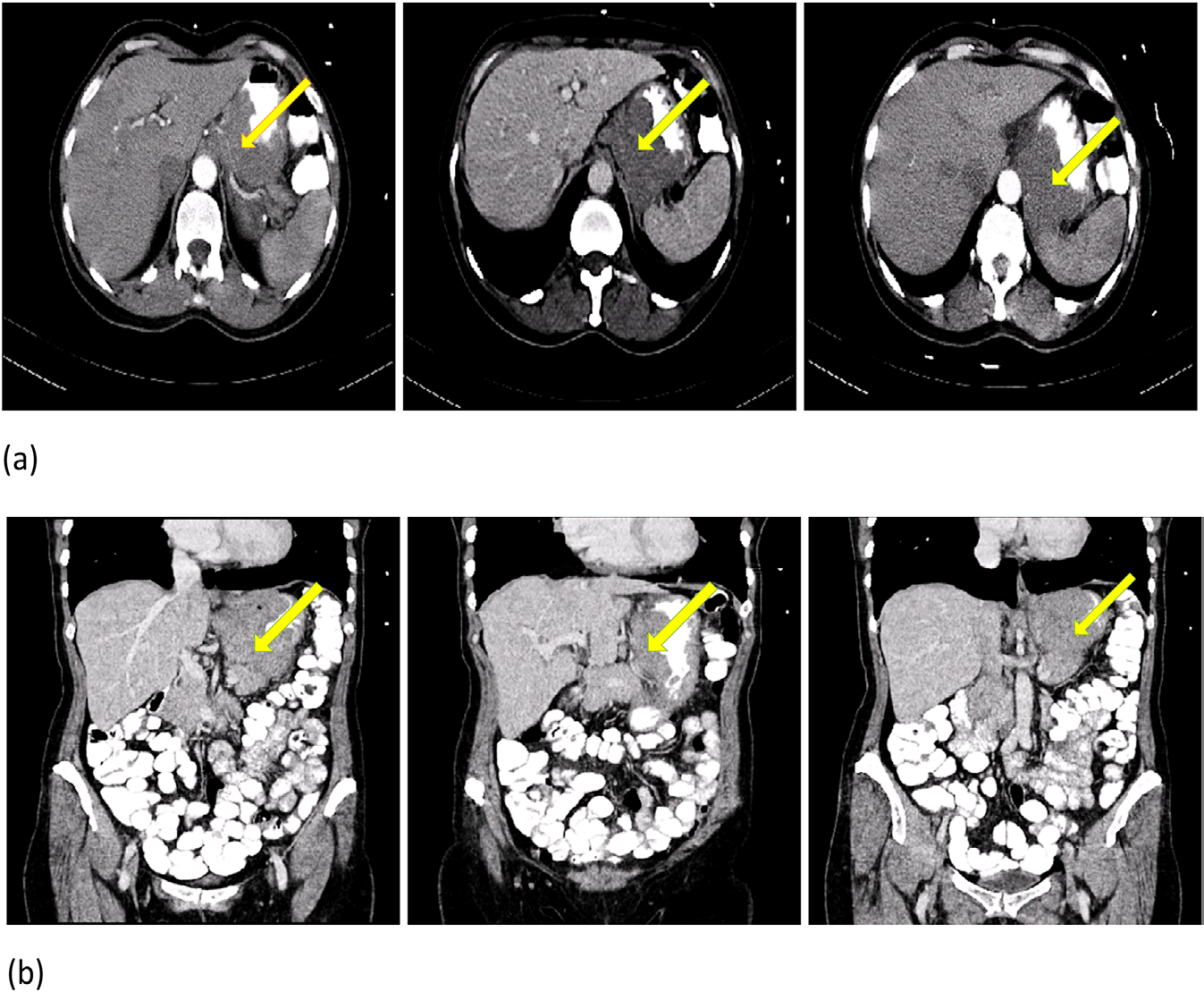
CT scan of the abdomen and the pelvis with and without injected and oral contrast after first admission. The yellow arrow shows a mass in the gastric fundus with extension to lesser curvature. (Panel A: Axial view. Panel B: Coronal view).
